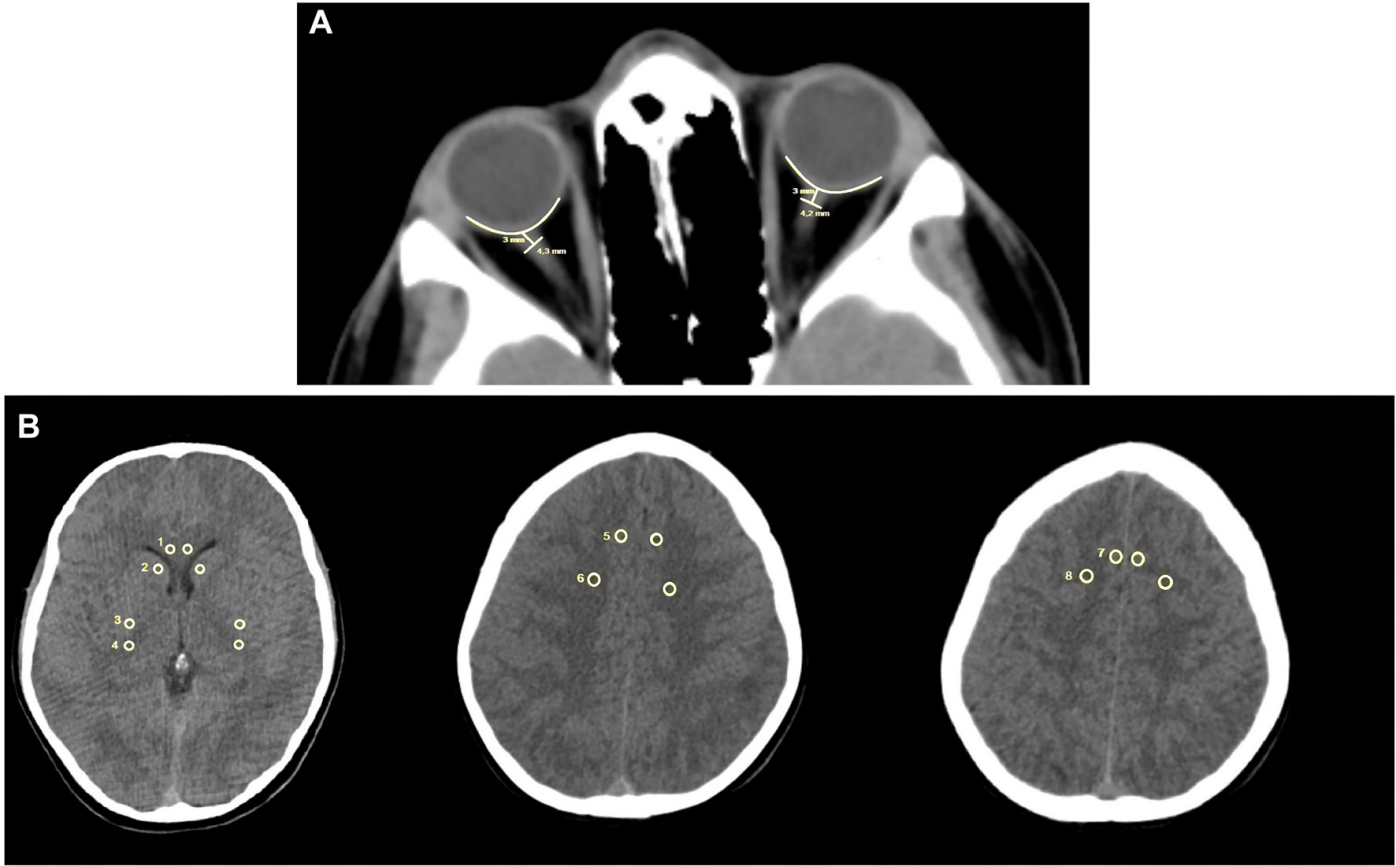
Two samples from patients’ CT records. (A) Measurement of ONSN with CT; (B) Measured areas at the upper cortex levels and basal ganglia level to calculate the mean GWR. GWR: Gray–white matter ratio; CT: Computed tomography; ONSD: Optic nerve sheath diameter.
